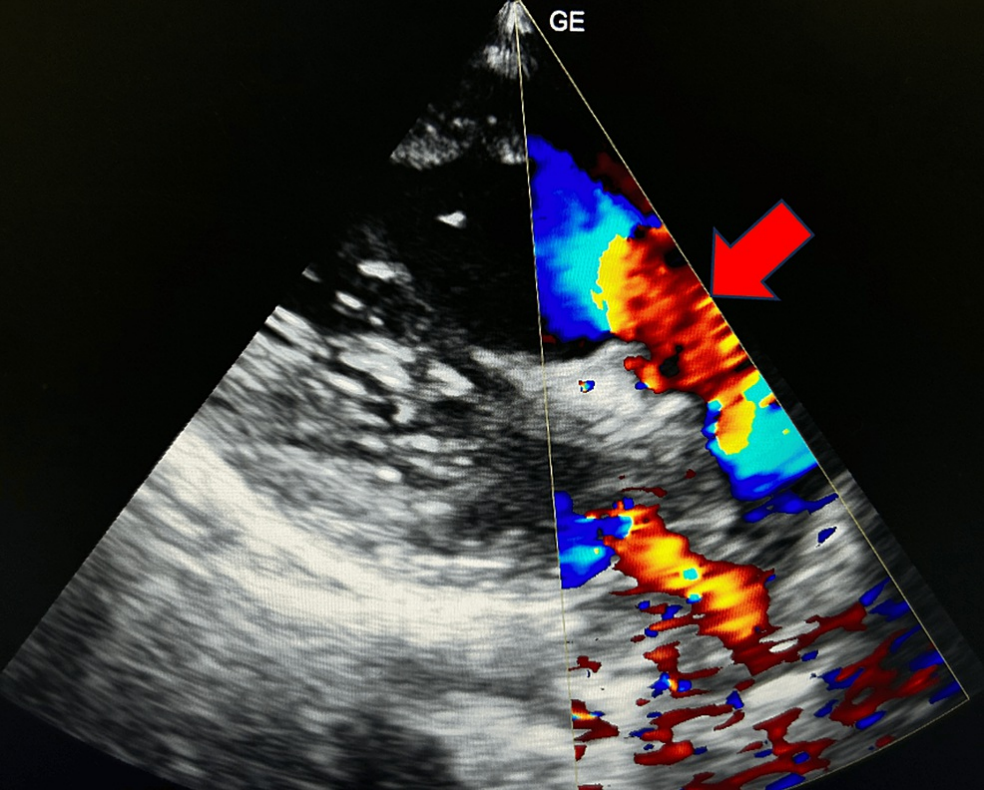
2D echocardiography PLAX view shows aorta arising from the right ventricle (red arrow). PLAX, parasternal long axis
